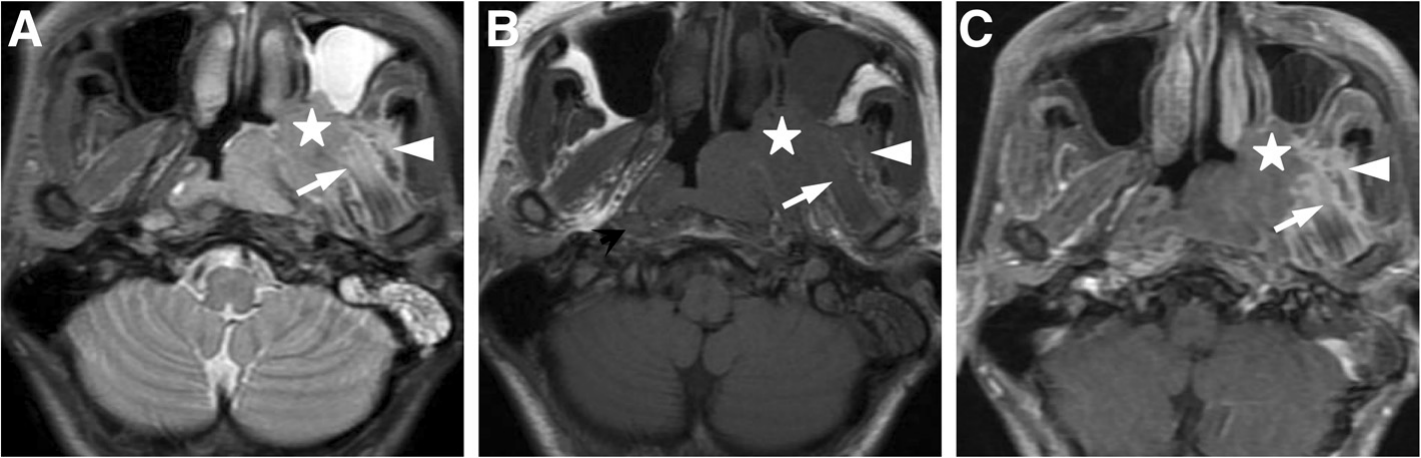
MR images of a 40-year old man with undifferentiated nonkeratinizing squamous cell nasopharyngeal carcinoma. MR images demonstrate NPC’s involvement of the MPM and LPM (long arrow), and the temporal muscle (arrowhead) as well as pterygopalatine fossa(star), which present a high signal intensity on axial PDWI image (a), a medium or low signal intensity on axial T1WI-TSE image (b), and an obvious enhancement on axial contrast-enhanced T1WI-TSE image with fat suppression (c). (MRI = Magnetic resonance imaging; NPC = Nasopharyngeal carcinoma; MPM = Medial pterygoid muscle; LPM = Lateral pterygoid muscle; PDWI = Proton density-weighted imaging; T1WI-TSE = T1 weighted imaging-Turbo spin echo)
